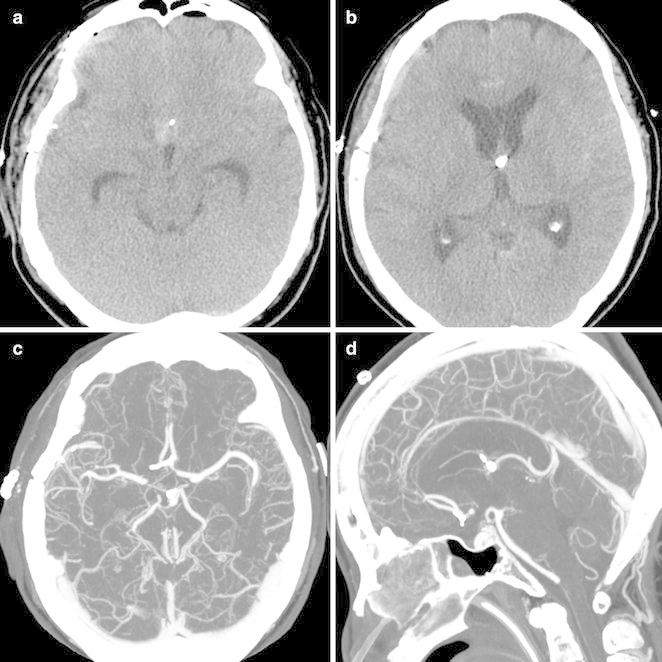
On post-operative Day 7, the hematoma was nearly absorbed and ambient cistern was visible (a). But ventricles were enlarged compared with the previous CT scan (b) and CT angiography demonstrated significant vasospasm at both distal portions of the anterior cerebral arteries (c, d)
